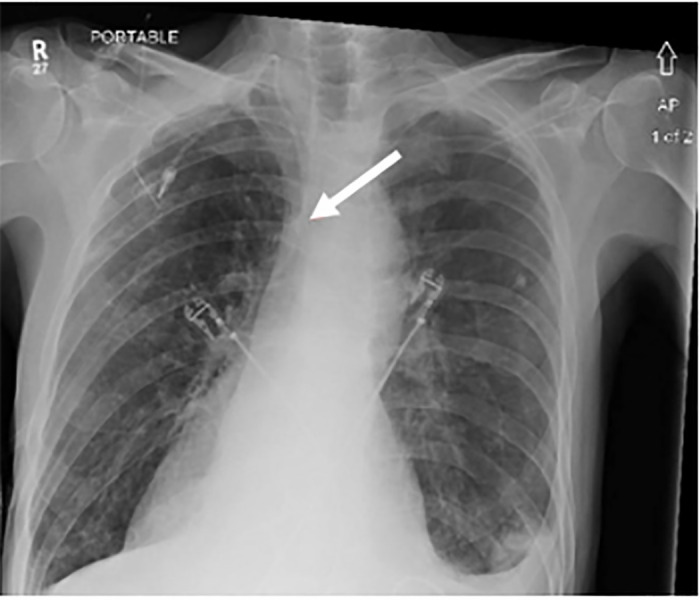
A chest radiograph depicting the right-sided chest port (arrow) with line
